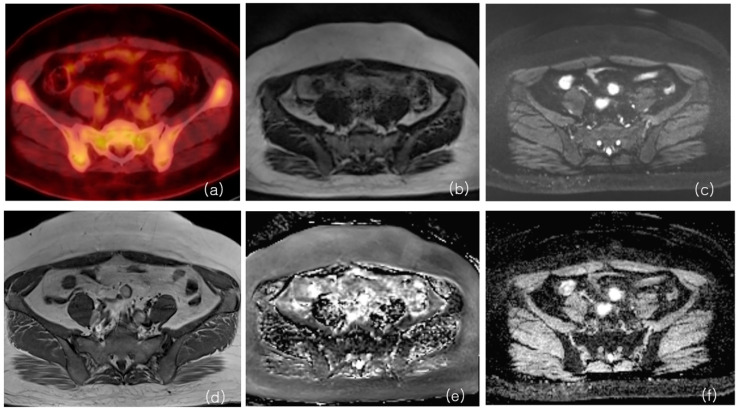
MRI and 18F-FDG PET/CT from a patient with red marrow hyperplasia. PET/CT shows a multifocal increased FDG uptake in bilateral iliac bone and sacrum (a). SUV peak from left iliac bone measured 3.15. The ADC max from BLADE b value was 800 DWI (b), and the ADC map (e) was 812. The ADC max from RESOLVE b value 800 DWI (c) and ADC map (f) was 454. T1-weighted non-contrast MRI (d) shows the bone marrow with diffuse intermediate SI, close to the signal intensity of muscle, compatible with red marrow.
